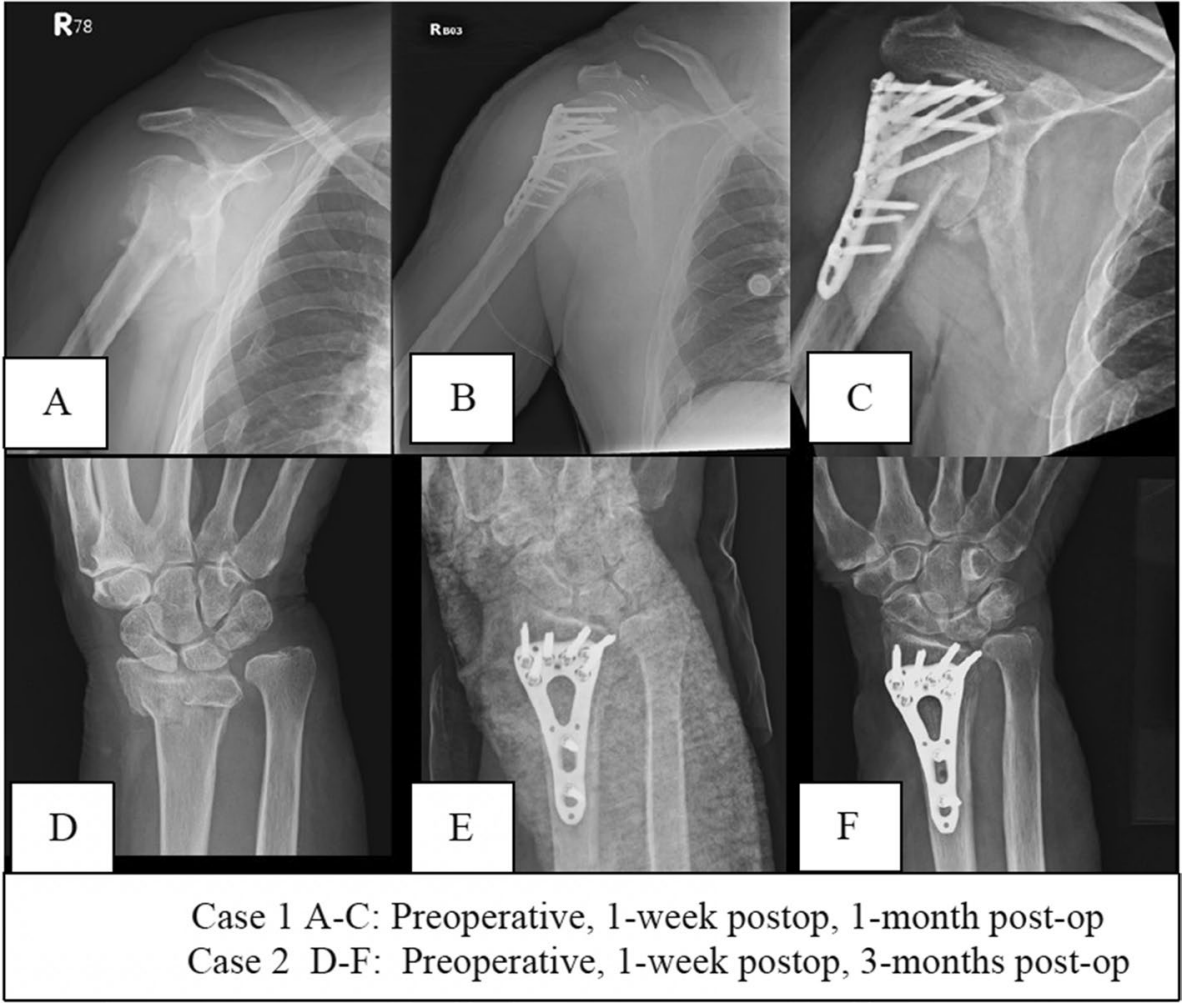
(A–C) This was a 75-year old male that presented with right proximal humerus fracture treated with AO-philos locking plate. Postop x-rays: revealed appropriate fixation and alignment. However, loss of reduction was noted 1 month after the surgery and eventually screw penetration was noted which required removal of implant at three months after the surgery. (D–F) A 67-year old female that received plating for a left distal radius fracture. Progressive loss of radial height and eventually screw penetration was noted, violating the wrist joint which required removal of implant.
